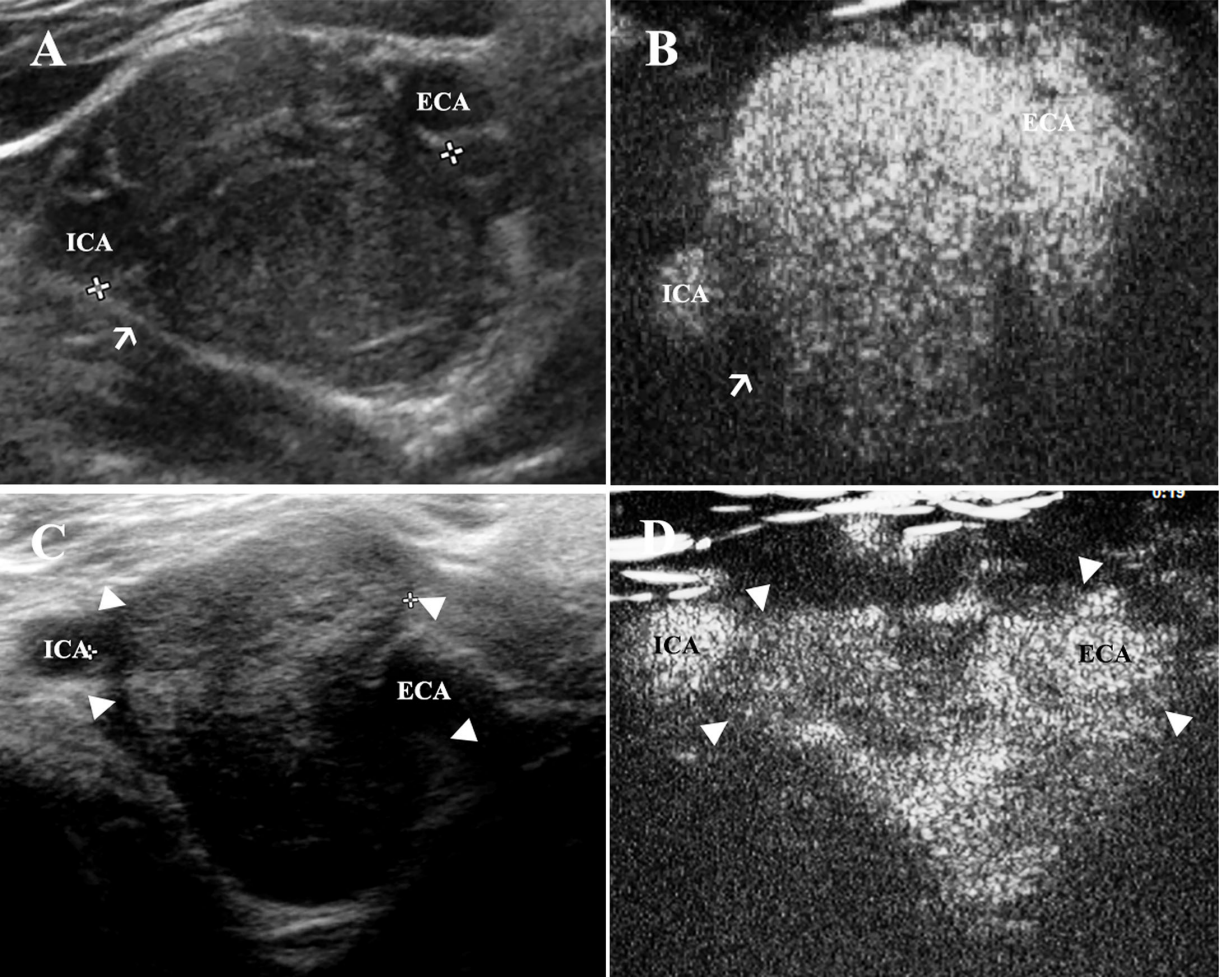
CEUS is superior to assess the morphology of CBT lesions in comparison to gray-scale ultrasound. (A) CBT lesions presented as oval-shaped and hypoechoic masses, which led to splaying of the carotid bifurcation. The adventitia of carotid vessels could be observed clearly on gray-scale ultrasound (thin arrow). (B) However, the adventitia (thin arrow) could hardly be visualized in CEUS during the artery phase. (C) A very small part of ICA was encased by the lesion area. The margin (triangles) of the tumor around ECA could not be seen clearly, and a Shamblin type II was identified using grey-scale ultrasound. (D) The margin of the tumor (triangles) could be better observed using CEUS and a Shamblin type III was reclassified. CBT, carotid body tumor; CEUS, contrast-enhanced ultrasonography; ICA, internal carotid artery; ECA, external carotid artery.
